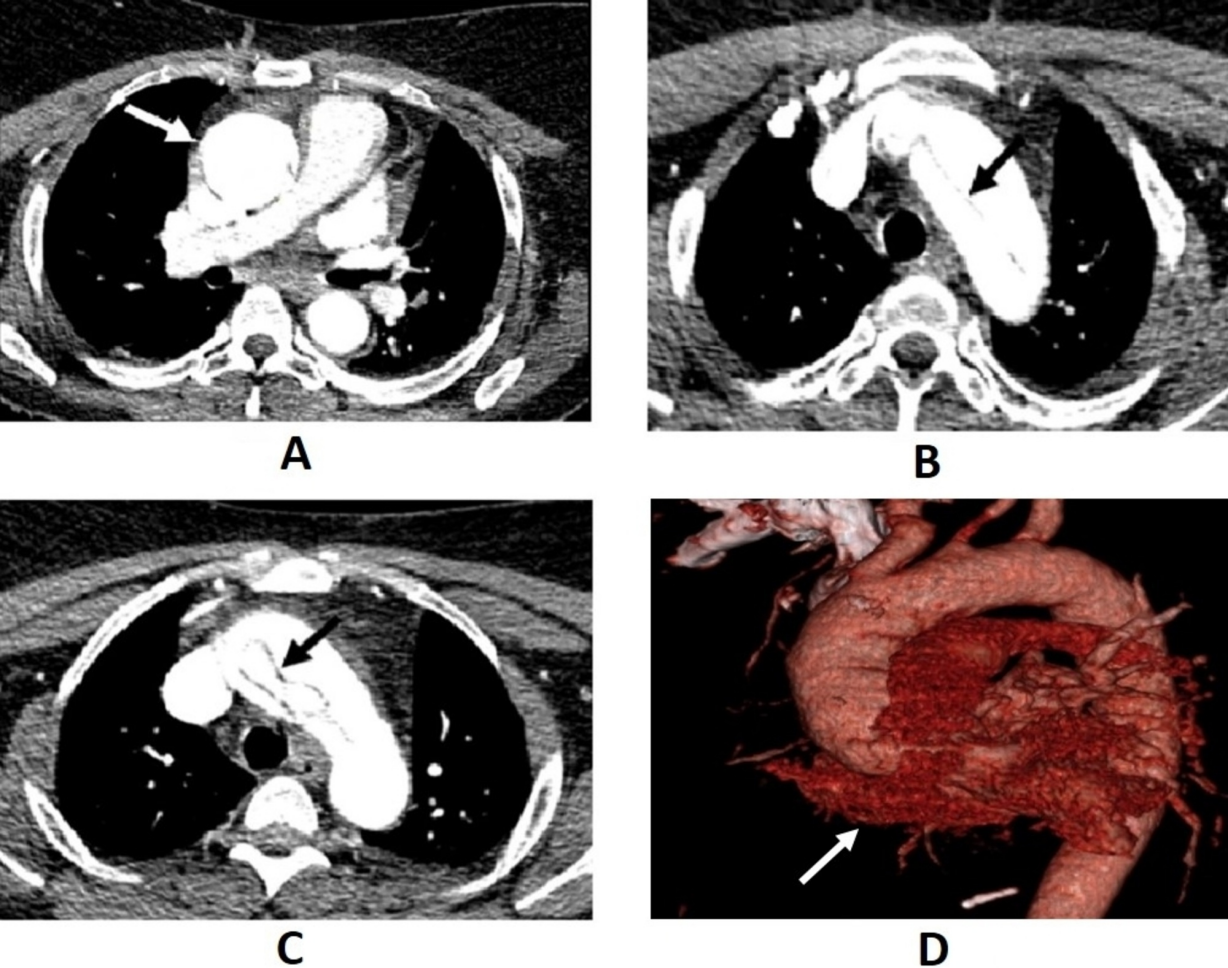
Computed tomography angiography showing (A) dilation of the ascending aorta with a diameter around 44 mm, (B and C) dissection of the ascending aorta, and (D) three-dimensional reconstruction of the aorta (with the false lumen in dark red).
